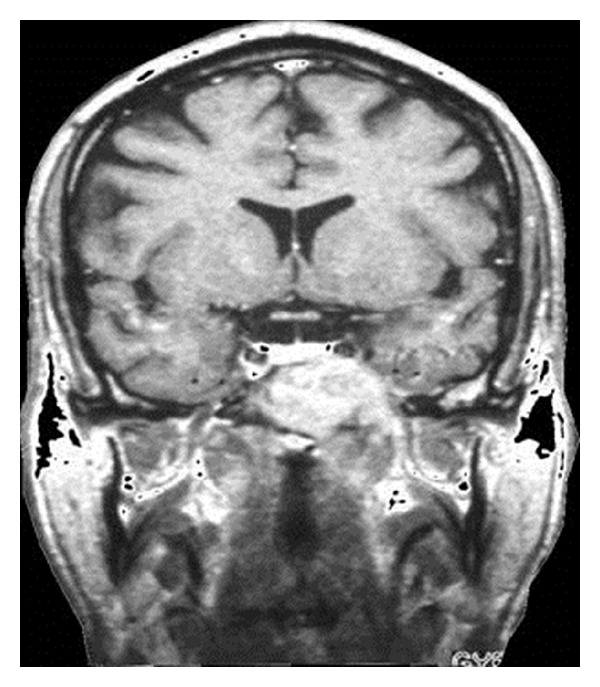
Cranial MRI before radiotherapy.
